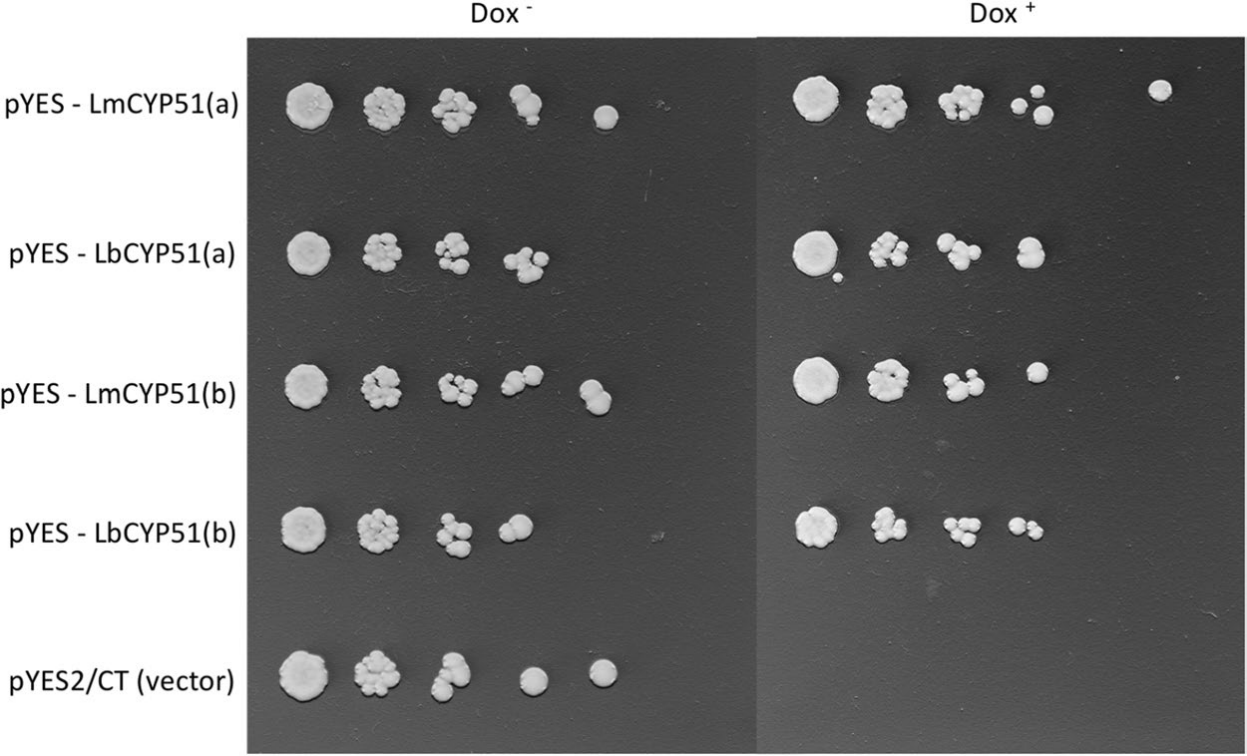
Complementation of *Saccharomyces cerevisiae* strain *YUG37::erg11* with *Leptosphaeria maculans* CYP51B and *Leptosphaeria biglobosa* CYP51B. Yeast transformants grown for 16 hours in SD + GAL + RAF media were inoculated onto SD + GAL + RAF agar without (DOX^−^) or with (DOX^+^) doxycycline, which represses the native *S. cerevisiae* CYP51B. The YUG37::pYES2/CT vector-only strain was used as a control. Lettering (a,b) refers to biological replicates.
